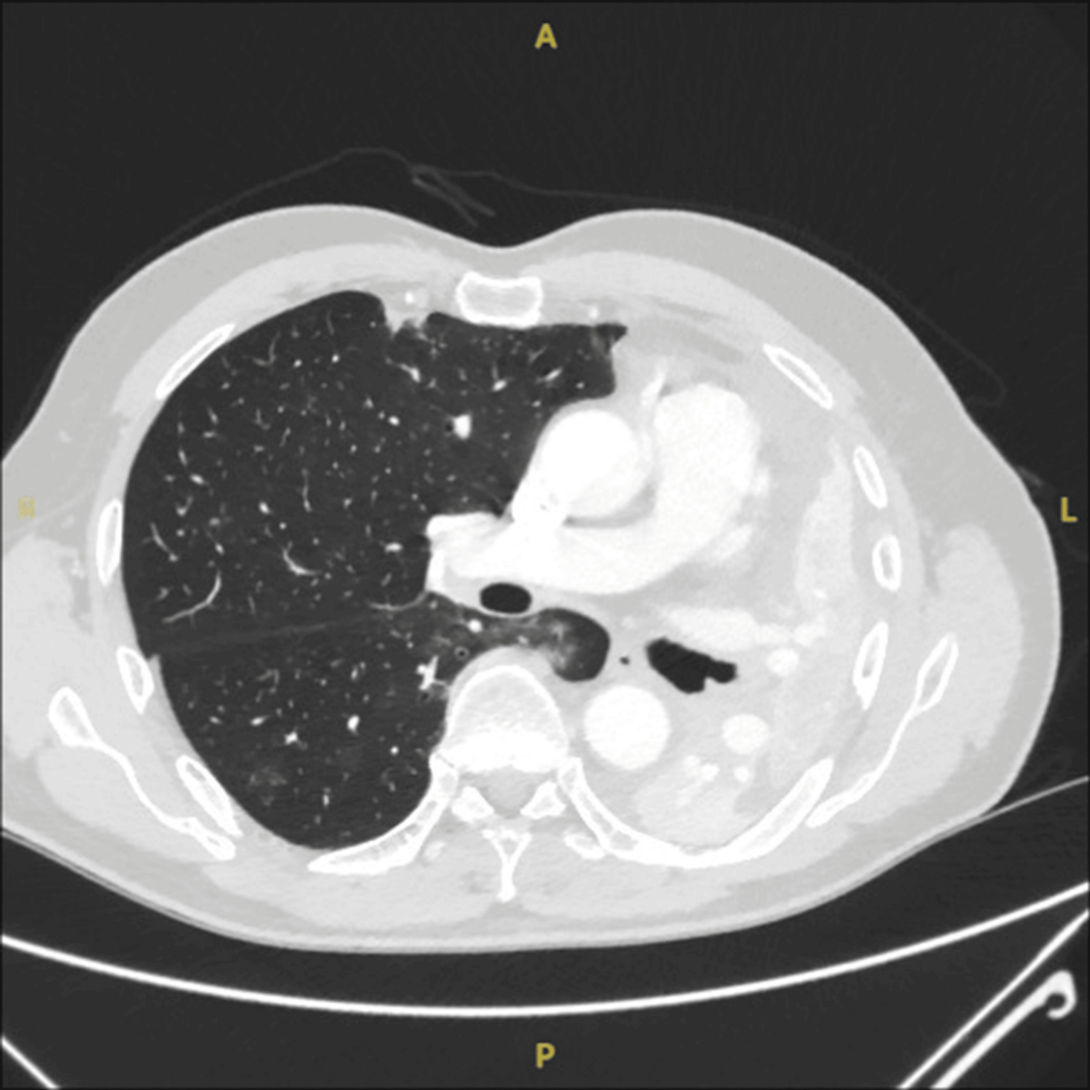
Slice of the CT scan just below the carina to show obstruction in the left main bronchus causing total left lung collapse.
